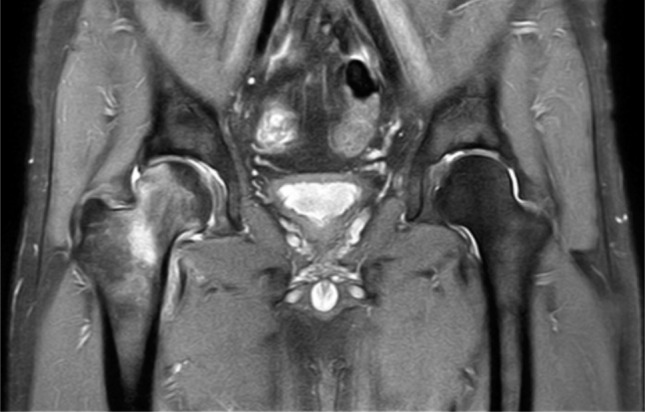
T2-weighted MRI showing high signal intensity on right femoral neck and head along with joint effusion.
